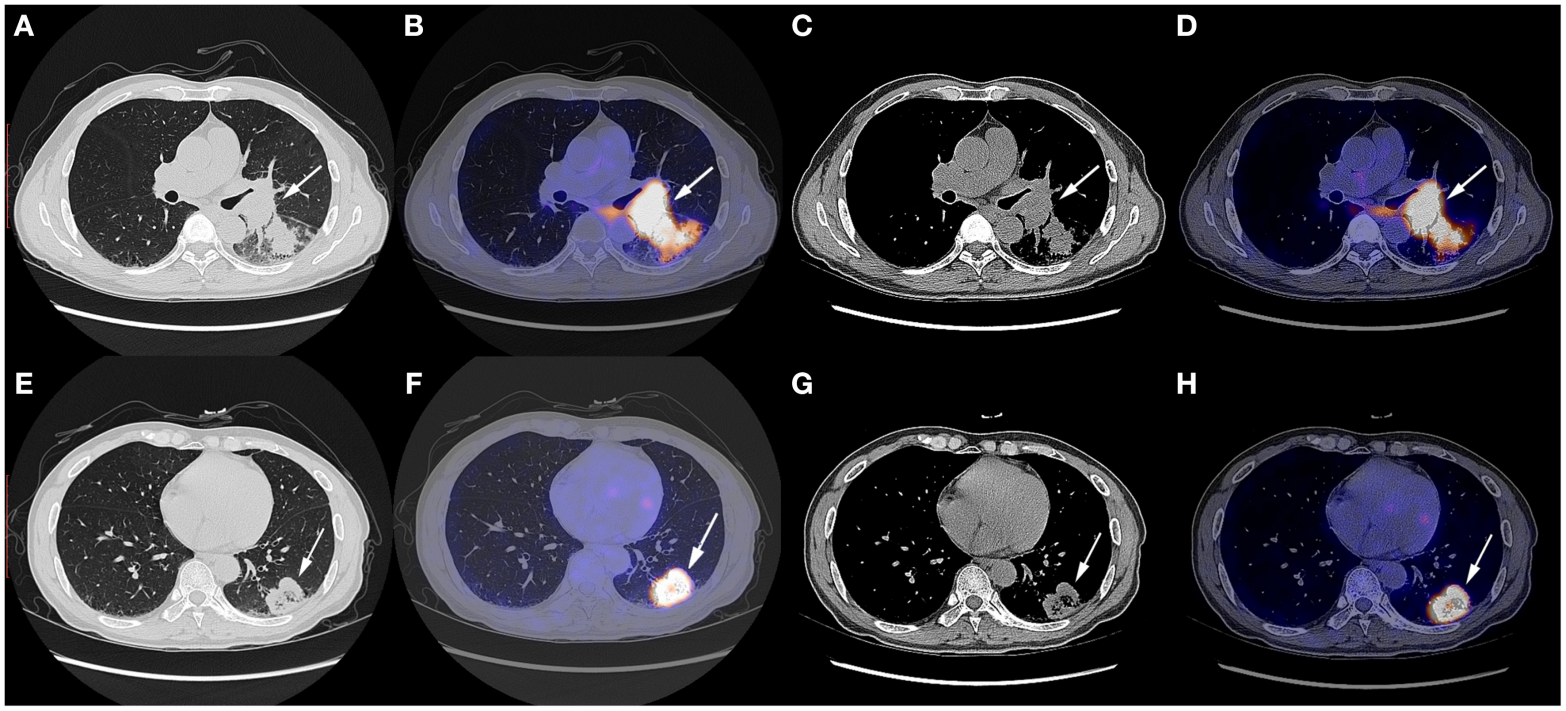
Chest CT scan and fused PET-CT images of the masses on October 9th, 2019. (A–D) The SUVmax of the mass adjacent to the hilum was 25.3 and the size of it was 3.6 cm × 5.2 cm × 4.8 cm; (E–H) The SUVmax of the mass on the basal segment was 12.5 and the size of it was 3.8 cm × 3.6 cm × 3.8 cm. White arrows indicate the locations of the masses.
